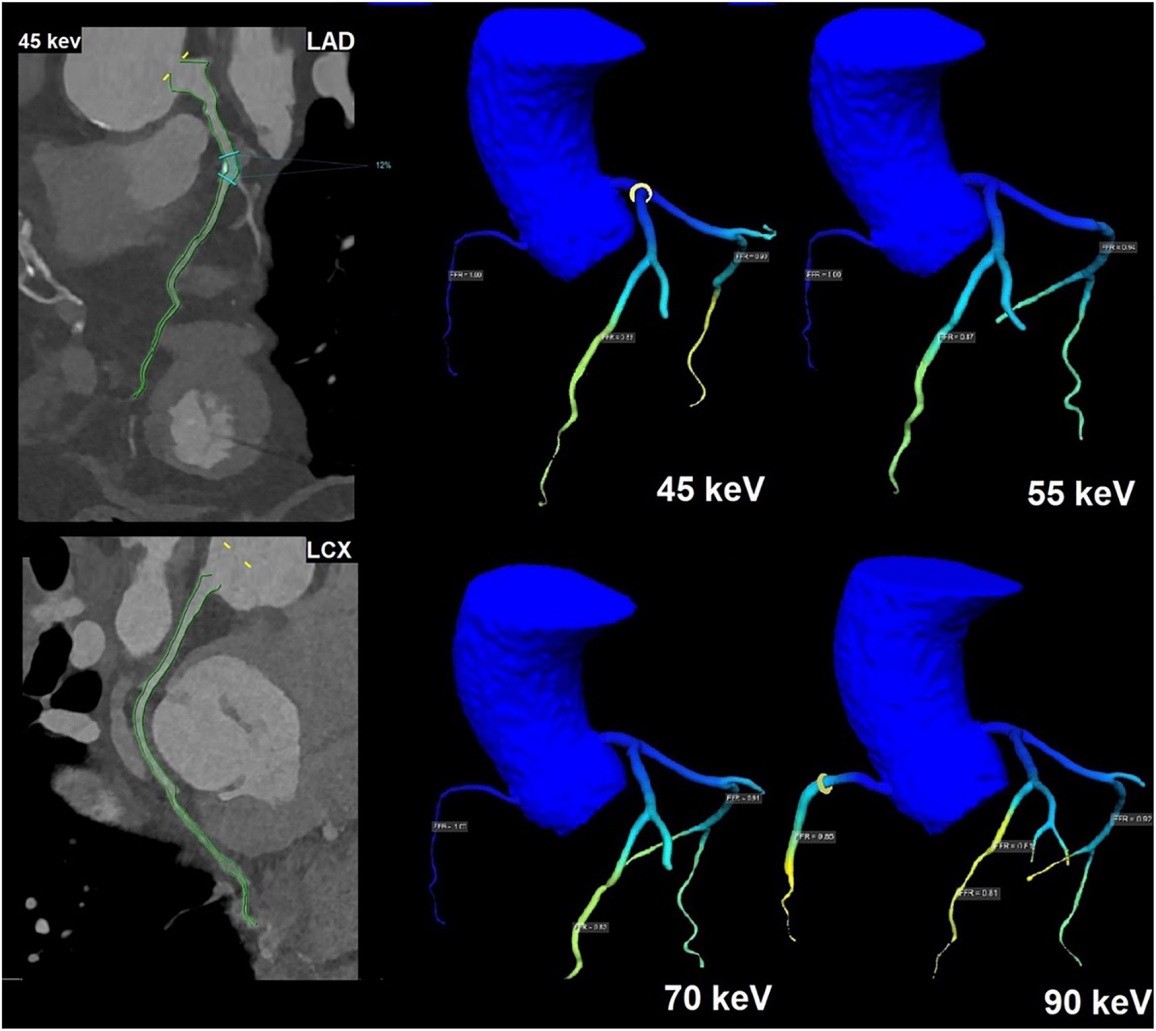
Image of a 66-year-old female patient with stenosis in the left anterior descending artery (LAD). Images are reconstructed at different virtual monoenergetic image (VMI) levels. Photon-counting detector (PCD)-CT images at 45 keV show a 12% LAD stenosis (marked by blue lines) by the machine learning–based software (cFFR prototype), and the final CT angiography-derived fractional flow reserve (CT-FFR) result. CT-FFR calculation shows no hemodynamical significance for the LAD (45 keV: 0.88, 55 keV: 0.87, 70 keV: 0.83, 90 keV: 0.81). No evidence of stenosis was found in the left circumflex artery and right coronary artery
